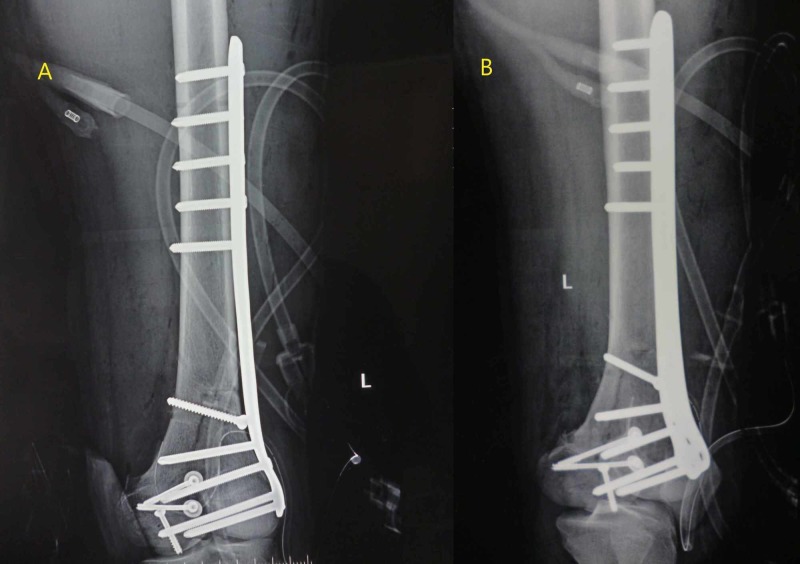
Immediate postoperative radiographs of the knee A: anteroposterior view; B: oblique view. The images show the reduction of the fracture with distal femur locking plate and screws
